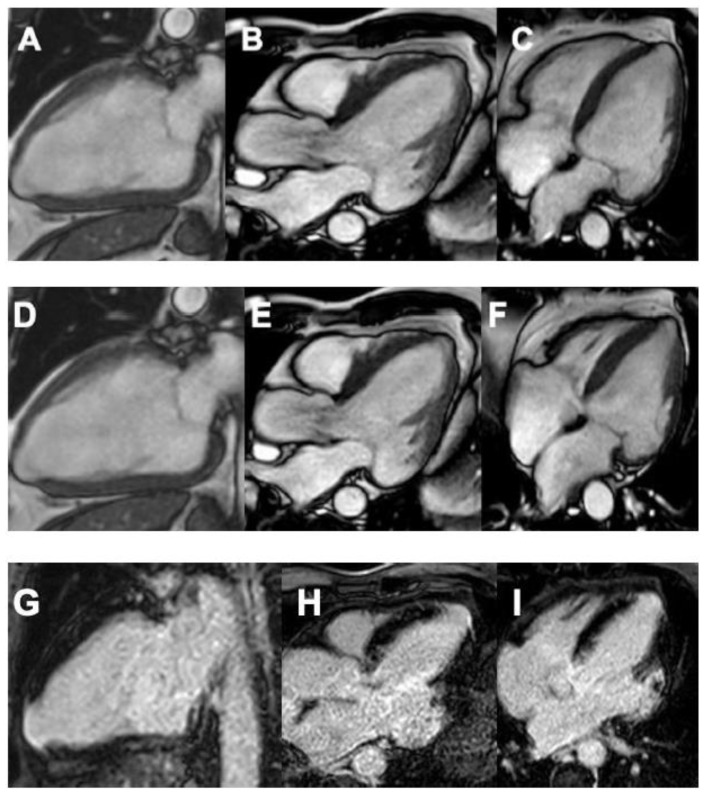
Apical and basal inferolateral aneurysm with the corresponding scar at the same location. (A–C): CMR cine end-diastolic frame in apical 2, 3, and 4 chamber views, (D–F): CMR cine end-systolic frame in apical 2, 3, and 4 chamber views, (G–I): CMR-LGE in apical 2, 3, and 4 chamber views.
